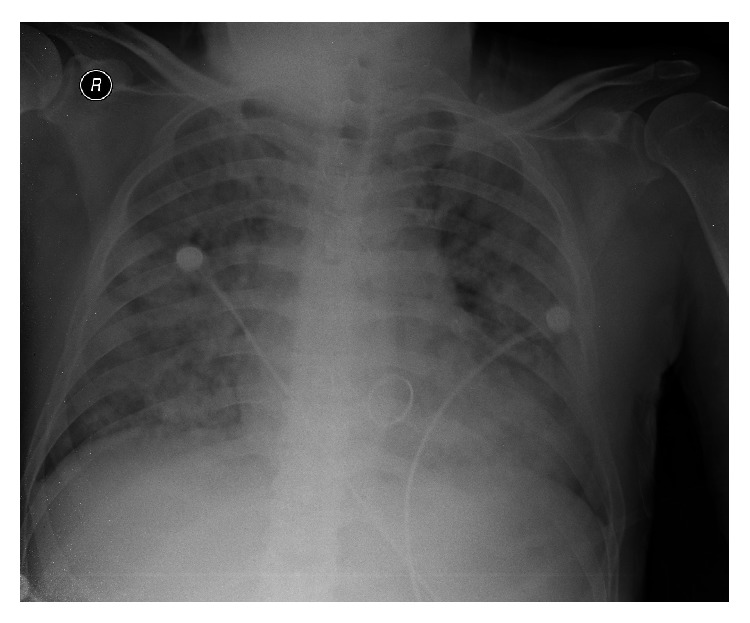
Chest X-ray on admission. Chest X-ray showed alveolar opacities in both lungs, especially in the perihilar and pericardiac zones.
